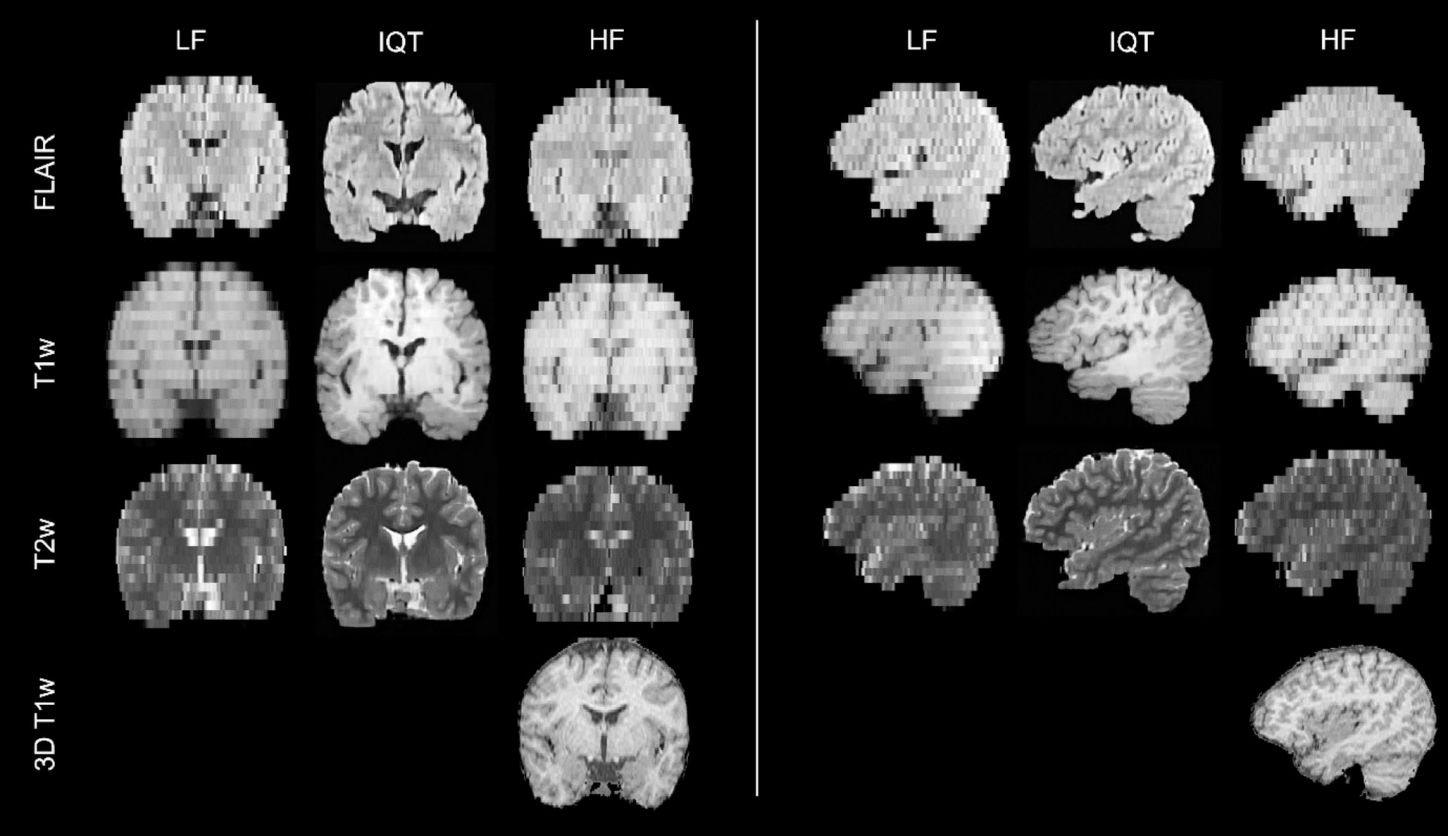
Representative coronal (left) and sagittal (right) reformatted images from a 13-year-old patient with lesion-negative brain MRI. The 3D T1w image acquired at HF is also reported in the last row as gold standard reference
